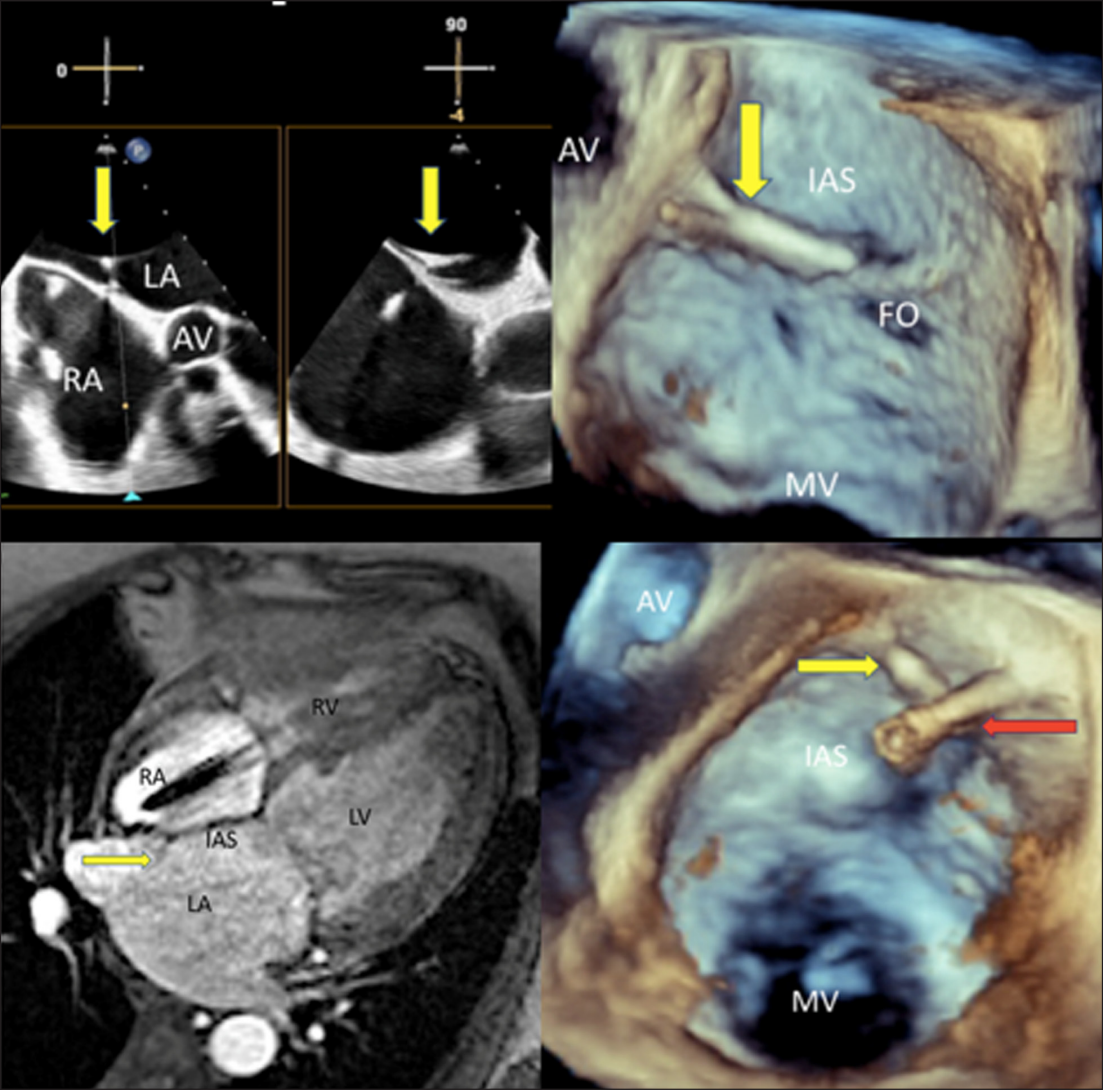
(A) Transesophageal echocardiogram (TEE) 2-dimensional (2D) imaging of the interatrial septum with the atrial septal ridge shown by the yellow arrows. (B) 3D TEE rotated view of the interatrial septum with the fossa ovalis shown in plane with the ridge (yellow arrow). (C) Cardiac magnetic resonance cine SSFP 4-chamber image demonstrating the atrial septal ridge (yellow arrow). (D) The MitraClip transcatheter mitral valve delivery system safely traversed across the interatrial septum (red arrow) with the yellow arrow pointing towards the atrial septal ridge. RA: right atrium; LA: left atrium; AV: aortic valve; IAS: interatrial septum; FO: fossa ovalis; MV: mitral valve
